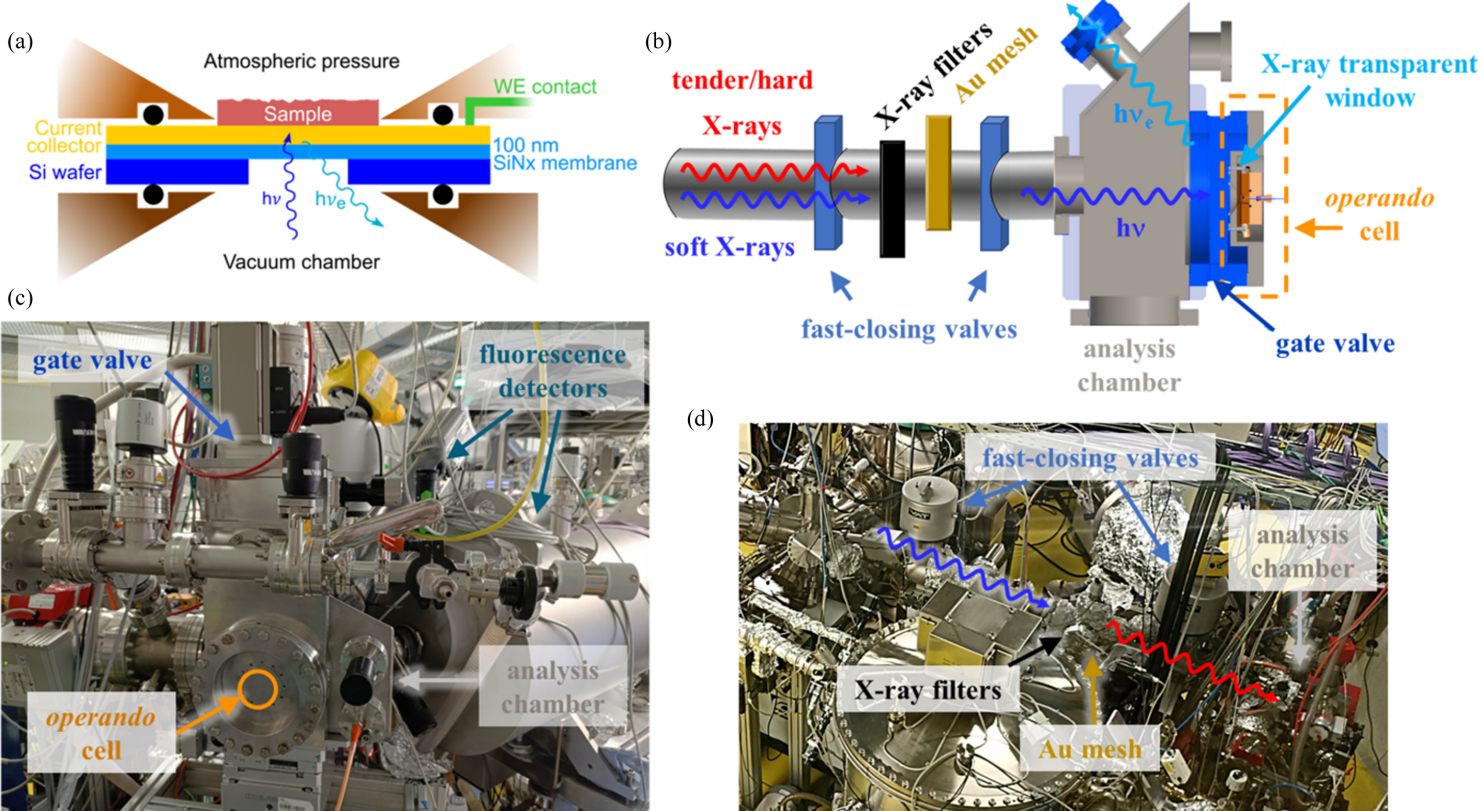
(*a*) Conceptual design of the sample environment built around a thin X-ray transparent window (100 nm thick SiN_*x*_ in this case). (*b*) Scheme of the endstation, including the sample environment, the analysis chamber and the *operando* beamline section. Photographs of (*c*) the analysis chamber and (*d*) the *operando* beamline section, where the most significant components discussed in the text are labelled.
